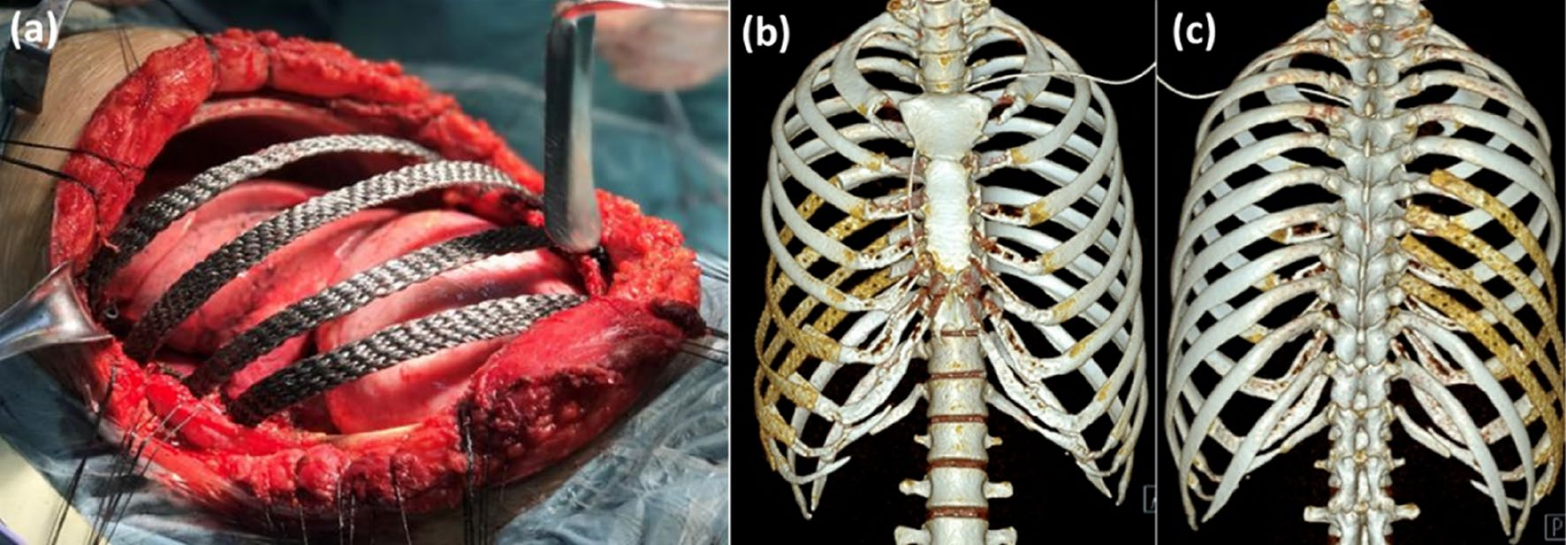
The right 6th to 9th ribs reconstruction at human implantation: (a) artificial rib implantation; postoperative CT image reconstruction, (b) anterior side; (c) rear side.
